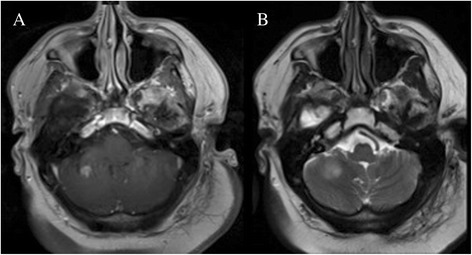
Imaging features of the recurrent tumor. a T1-weighted post-contrast and b T2-weighted MRI demonstrated an enhancing lesion in the right cerebellum with peri-lesional edema
